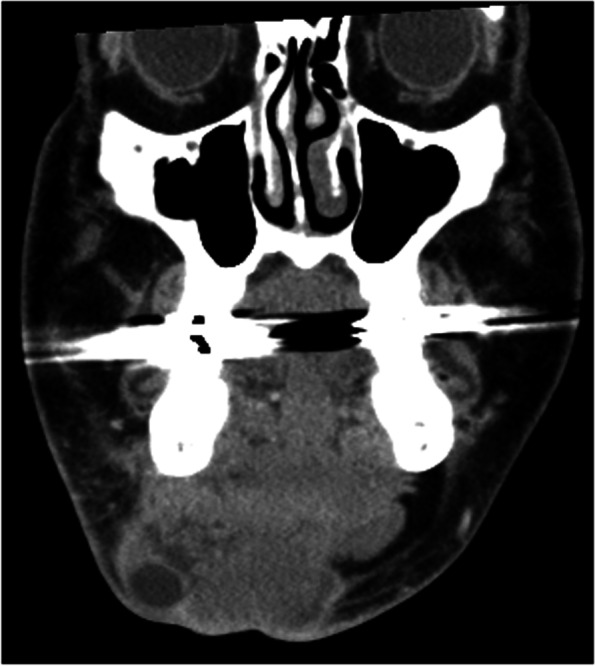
Coronal computed tomographic images of first recurrence indicated that the recurrent lesion was infiltrated into right submandibular glands and involving enlarged lymph nodes with peripheral rim enhancement
